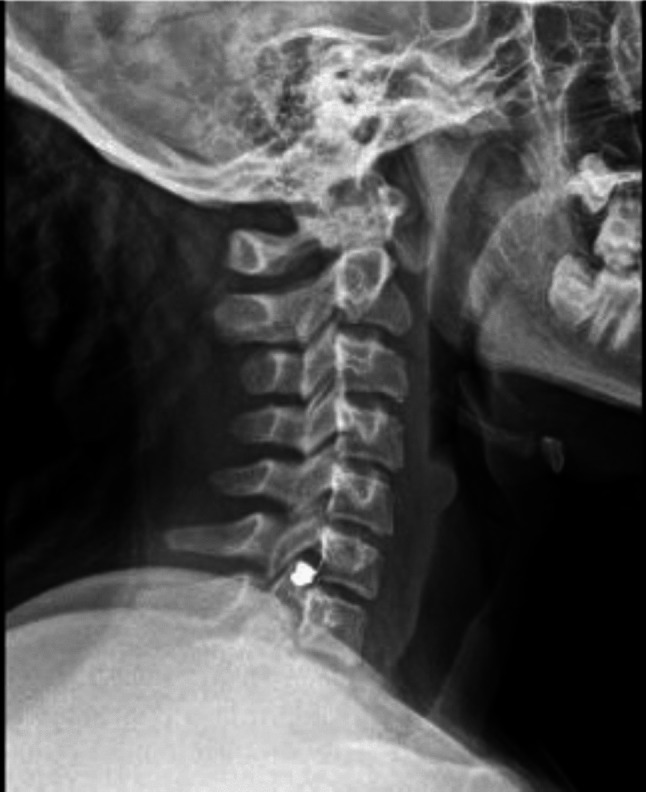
A lateral cervical X-ray reveals the ballistic fragment at the level of the C6–C7 vertebrae
